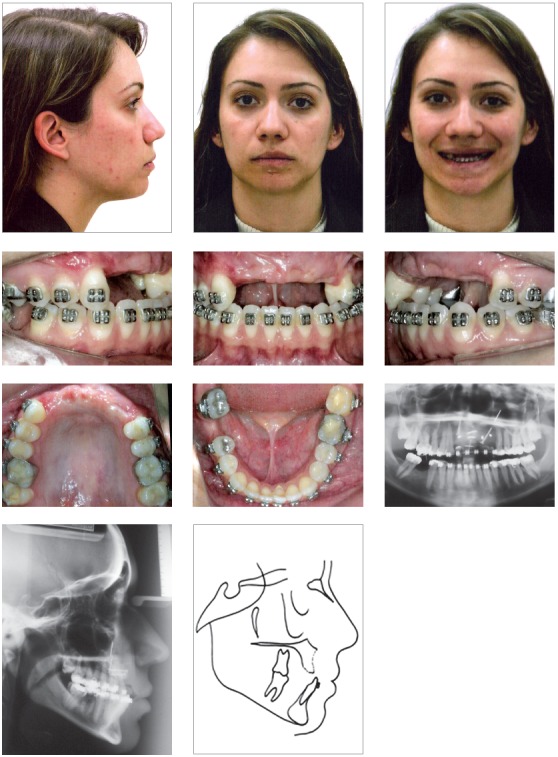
Intraoral photographs following placement of the alveolar bone graft. Panoramic radiograph showing the positioning of the alveolar bone graft.
